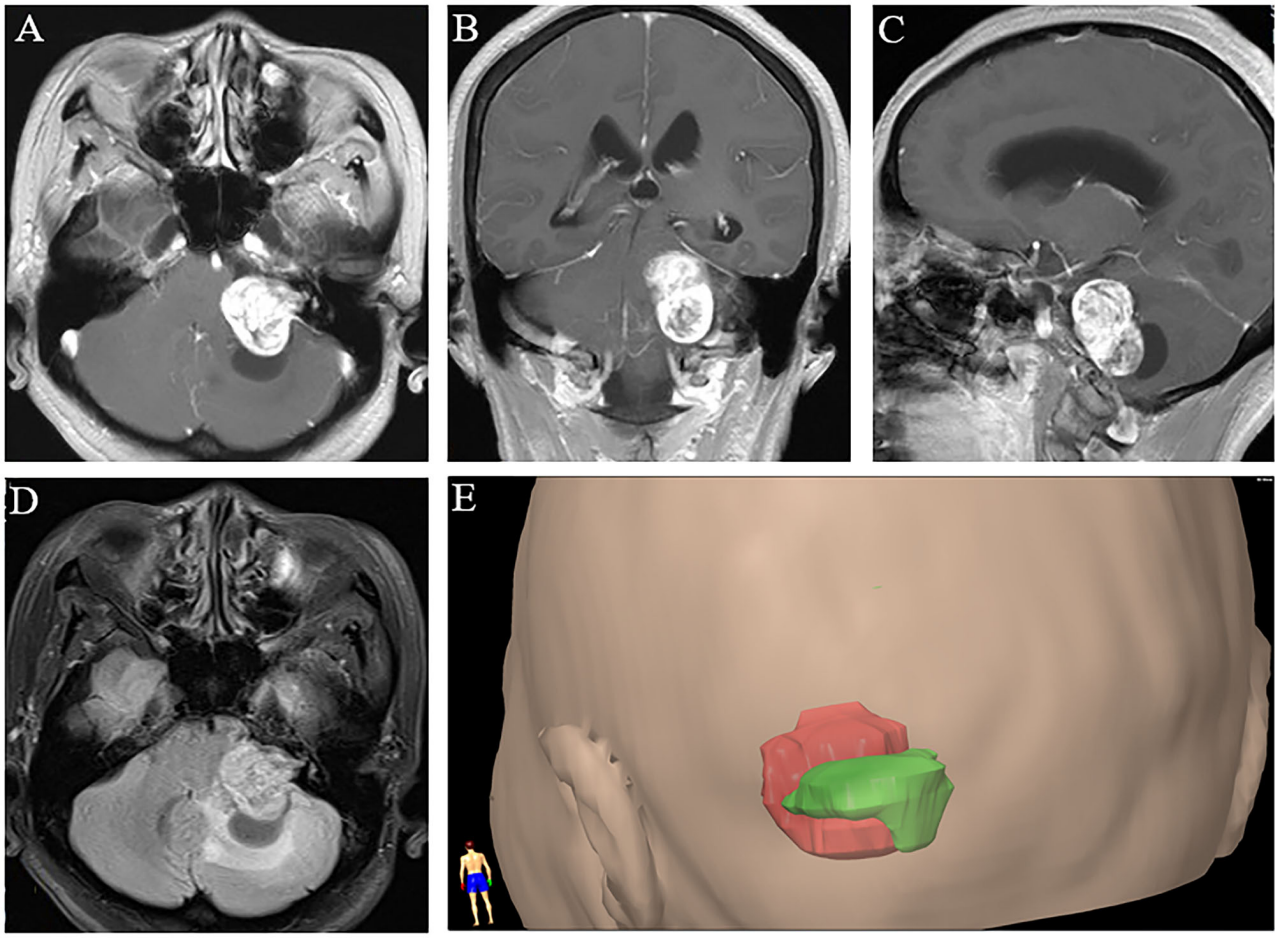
Three-dimensional magnetic resonance imaging reconstruction of vestibular schwannoma with peritumoral brain edema. (A–C) Post-contrast axial, coronal, and sagittal T1-weighted images. (D) T2-weighted fluid-attenuated inversion-recovery image. (E) A representative image of three-dimensional reconstruction with tumor in red and peritumoral edema in green.
